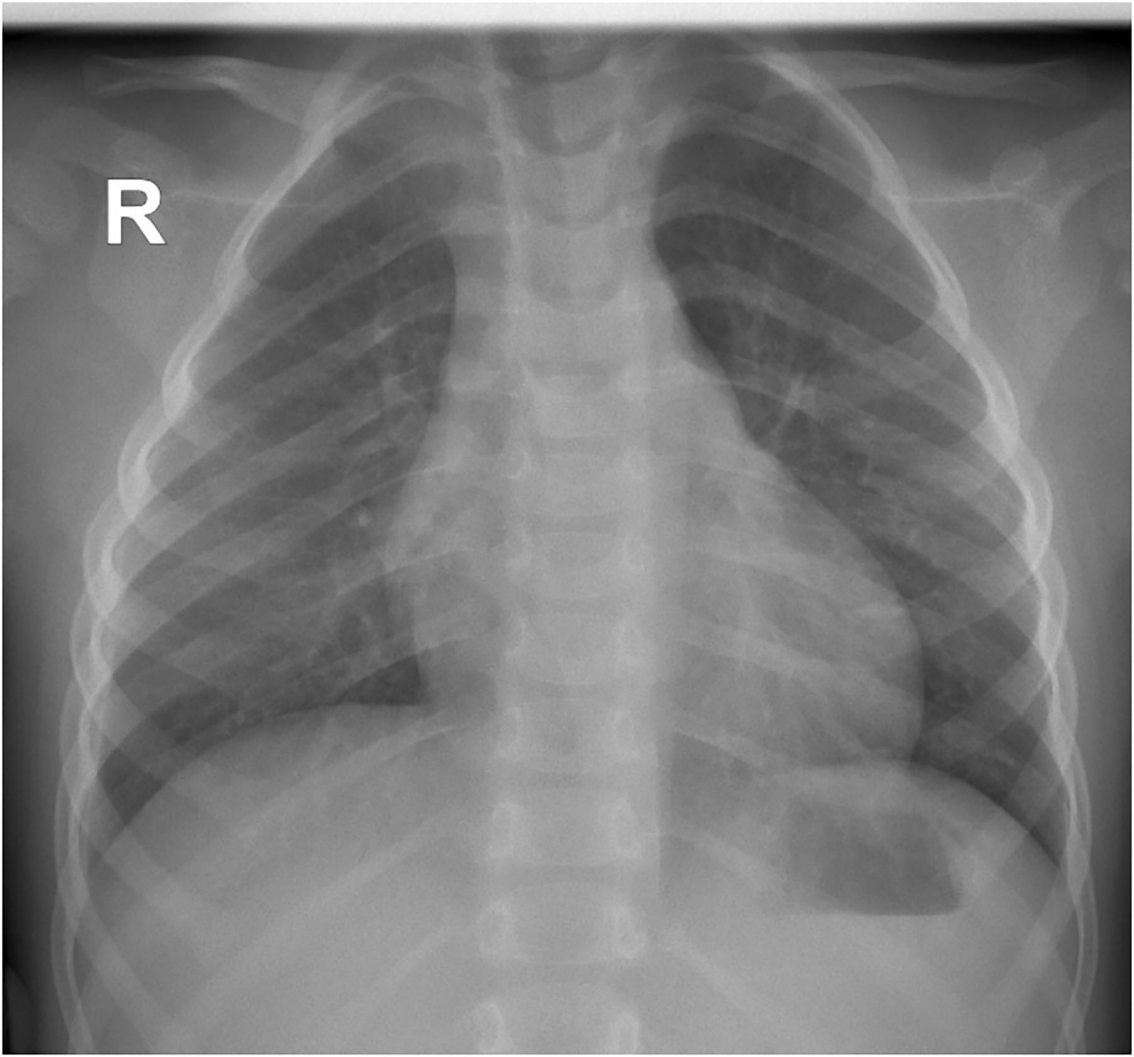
Thoracic radiography after 6 months revealing only minimal thickening of the inferior right pleura.
